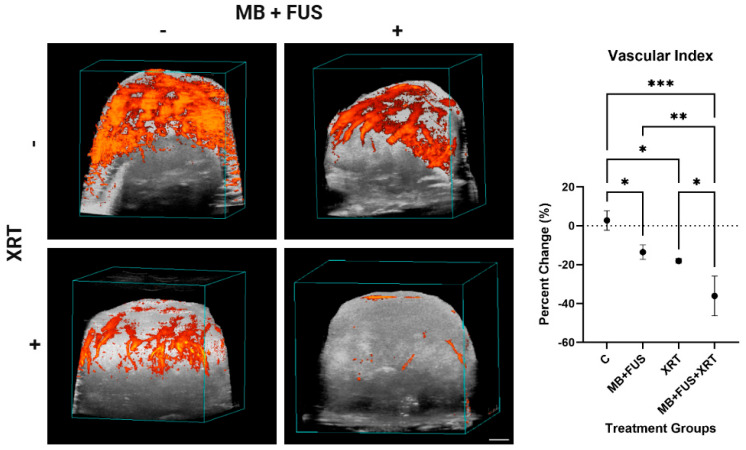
In vivo assessment of tumour perfusion using 3D Power Doppler (PD) ultrasound imaging. Left panel: Representative volumetric reconstructions of tumour vasculature (orange) are shown for each treatment group. Vascularity is visualized in a 3D overlay on B-mode grayscale anatomical images. Scale bar: 2 mm. Right panel: Average Vascular index (VI) across control (C), microbubble-enhanced focused ultrasound (MB + FUS), single-dose radiation therapy (XRT, 8 Gy), and MB + FUS + XRT treatment groups. Data are presented as mean ± SD. *p*-values ranging from *p* < 0.05 (*), *p* < 0.01 (), *p* < 0.001 (*).
